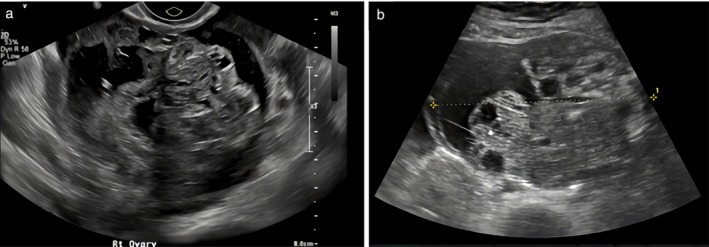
(a,b) Grayscale ultrasound images of two pure immature teratomas of the ovary. Using pattern recognition, these tumors were described as cystic tumors with solid components that seemed to arise from the cyst wall, but did not protrude into the cyst cavity like a papillary projection would. Solid components show pathognomonic echogenicity, with hyperechogenic areas, cysts and shadowing.
